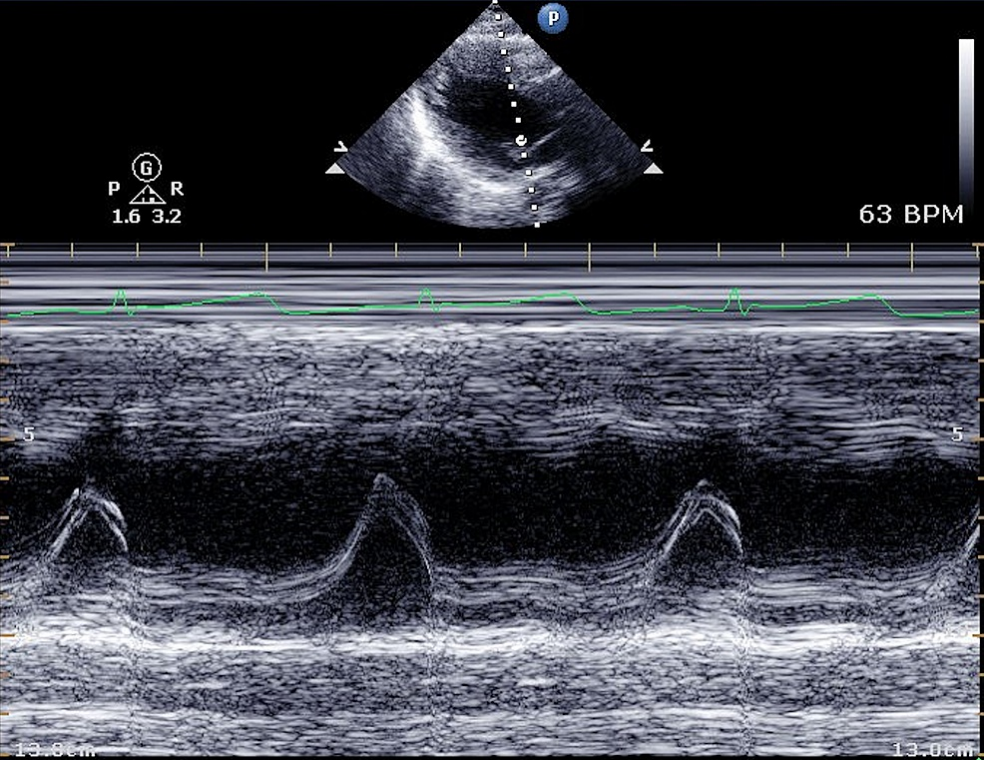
Initial echocardiogram showing M-mode through the mitral valve
